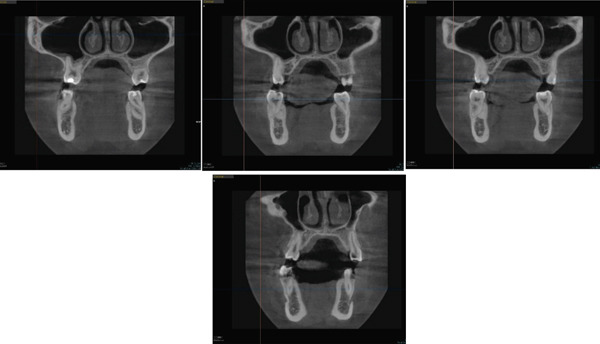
A CBCT 2 years posttreatment.
